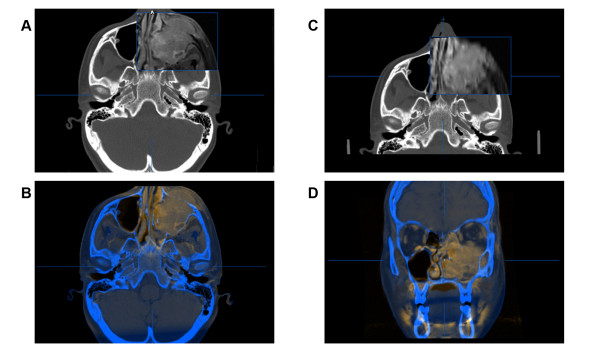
Fusion of initial CT scan data and MRI. (A+C) Window shows part of the MRI overlapping the initial CT scan. Both image modalities are shown simultaneously in transversal (B) and coronal (D) view.
